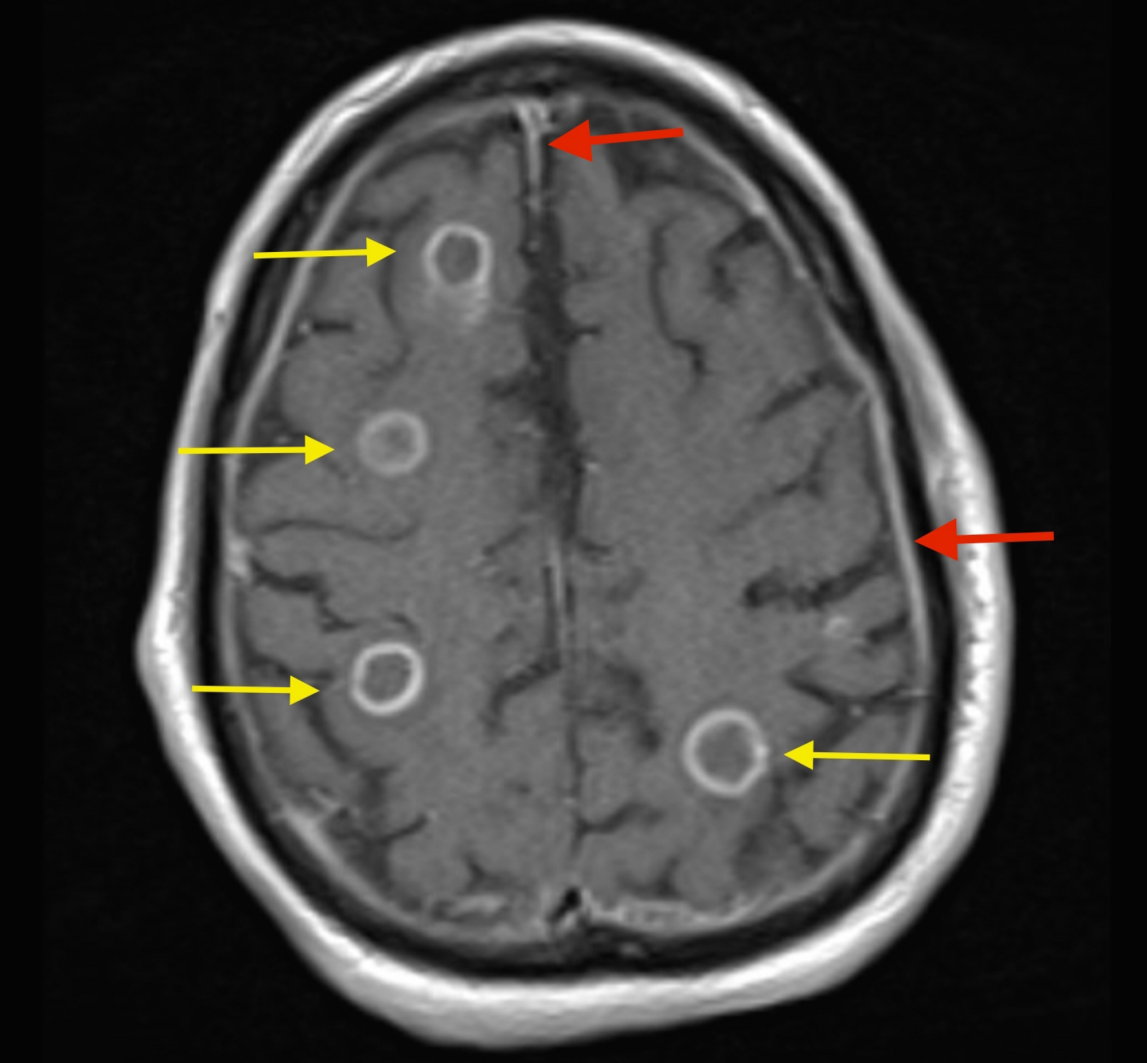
Magnetic resonance imaging (MRI) of the brain with contrast showing numerous ring enhancing lesions throughout brain parenchyma (yellow arrows) with meningeal enhancement (red arrows).
